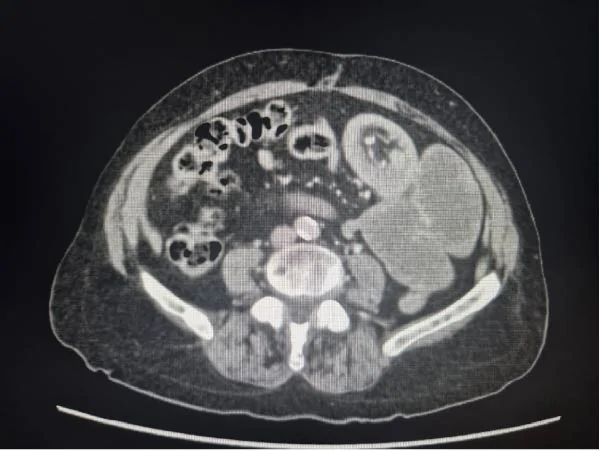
Axial slice of emergency CT abdomen pelvis demonstrating distended small bowel and two concentric hyperdense rings. Consistent with an intussusception.
